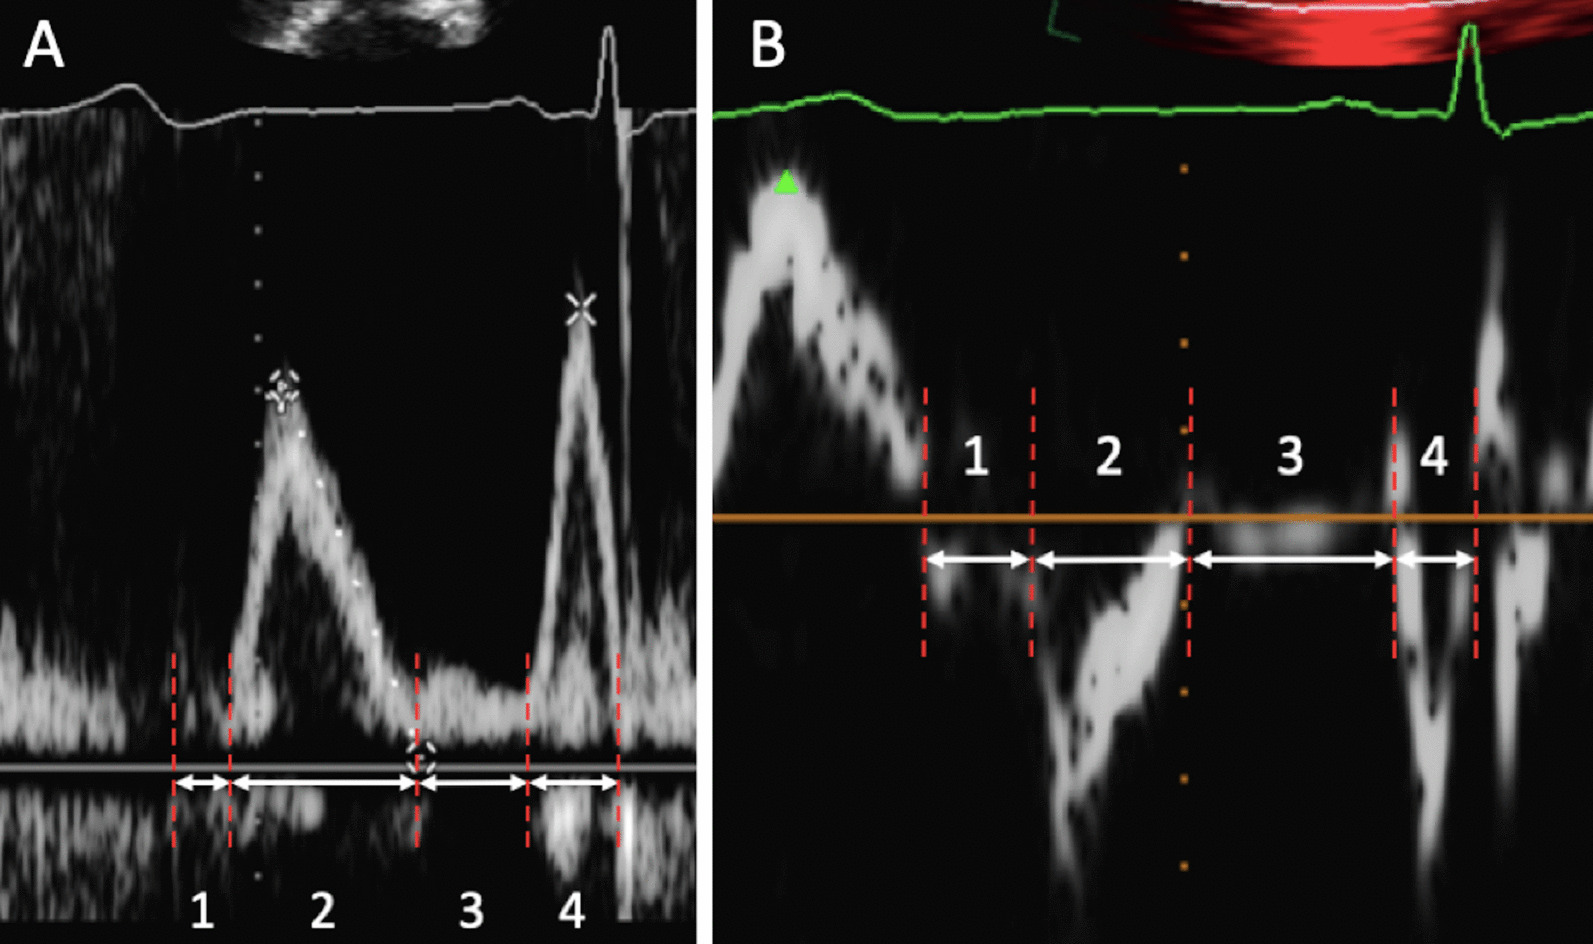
The four phases of diastole shown on a spectral Doppler trace of mitral inflow (A) and mitral annular tissue-Doppler imaging (B)—(1) IVRT, (2) early filling, (3) diastasis, (4) late filling from atrial contraction
